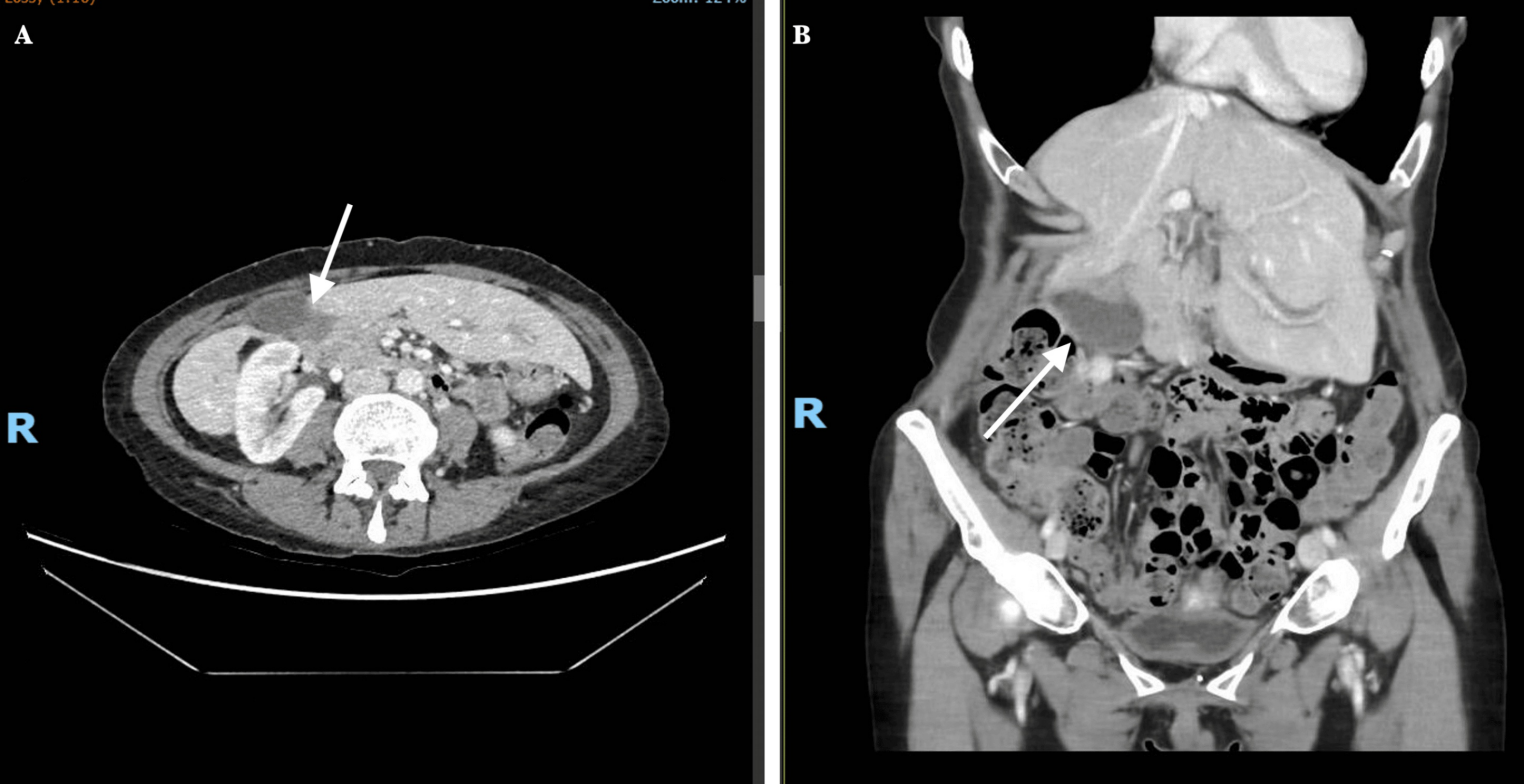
Repeat CT scan of gallbladder during the second presentation Repeat scan contrast-enhanced computed tomography (CT) in axial (A) and coronal (B) planes demonstrating mild pericholecystic inflammatory changes of the gallbladder (arrows) without evidence of acute abnormality, biliary dilation, or perforation.
